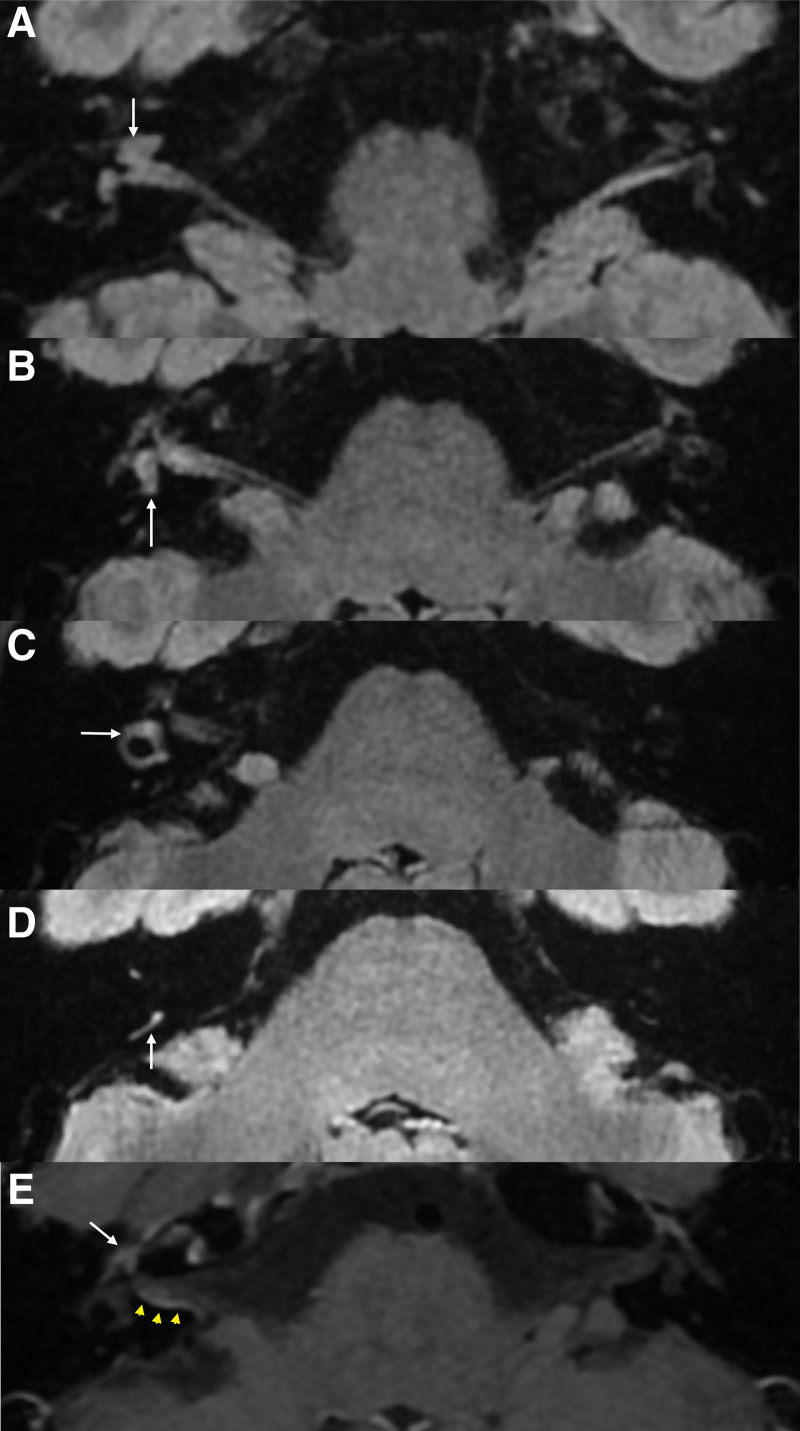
Four-hour-delayed postcontrast FLAIR temporal bone MRI demonstrated enhancement of the right cochlea (A, white arrow), the right vestibule (B, white arrow), the right lateral semicircular canal (C, white arrow), and the right posterior semicircular canal (D, white arrow). (E) The right facial nerve (white arrow) does not show more enhancement than the left facial nerve, and the dura along the posterior wall of the internal auditory canal (yellow arrowheads) is enhanced in postcontrast T1-weighted image. FLAIR = fluid attenuated inversion recovery, MRI = magnetic resonance imaging.
